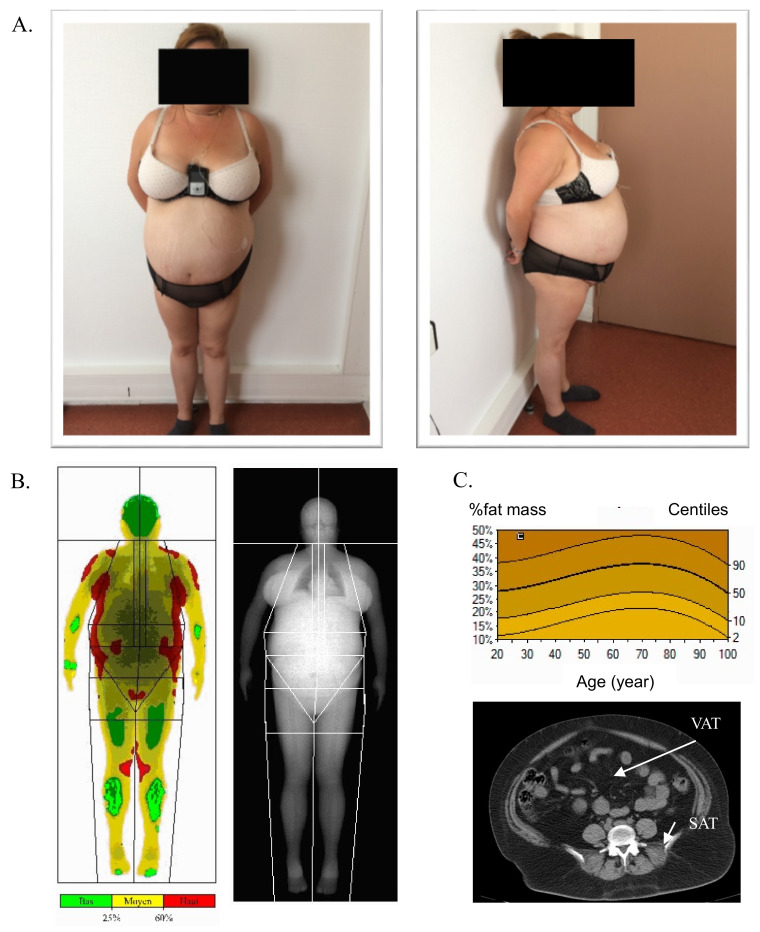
Patient clinical description. (A) Photographs of the patient showing central/android shape obesity with fat accumulation in the facial and supraclavicular regions and abdominal region and fat loss in lower limbs (cushingoid morphotype). (B) Dual energy X-ray absorptiometry (DEXA) showing body fat distribution. (C) Percentage of fat mass compared with the reference curve and abdominal computed tomography (CT) scan confirming the presence of an excessive accumulation of visceral adipose tissue (VAT) and subcutaneous adipose tissue (SAT).
